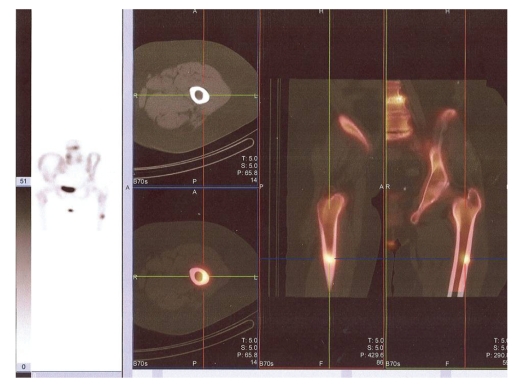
SPECT of the lesion on the left femur showing bilaterally thickened cortices and both internally and externally intact cortices. On the left side the bone scan showing a hotspot on the lateral side of the left femur.
